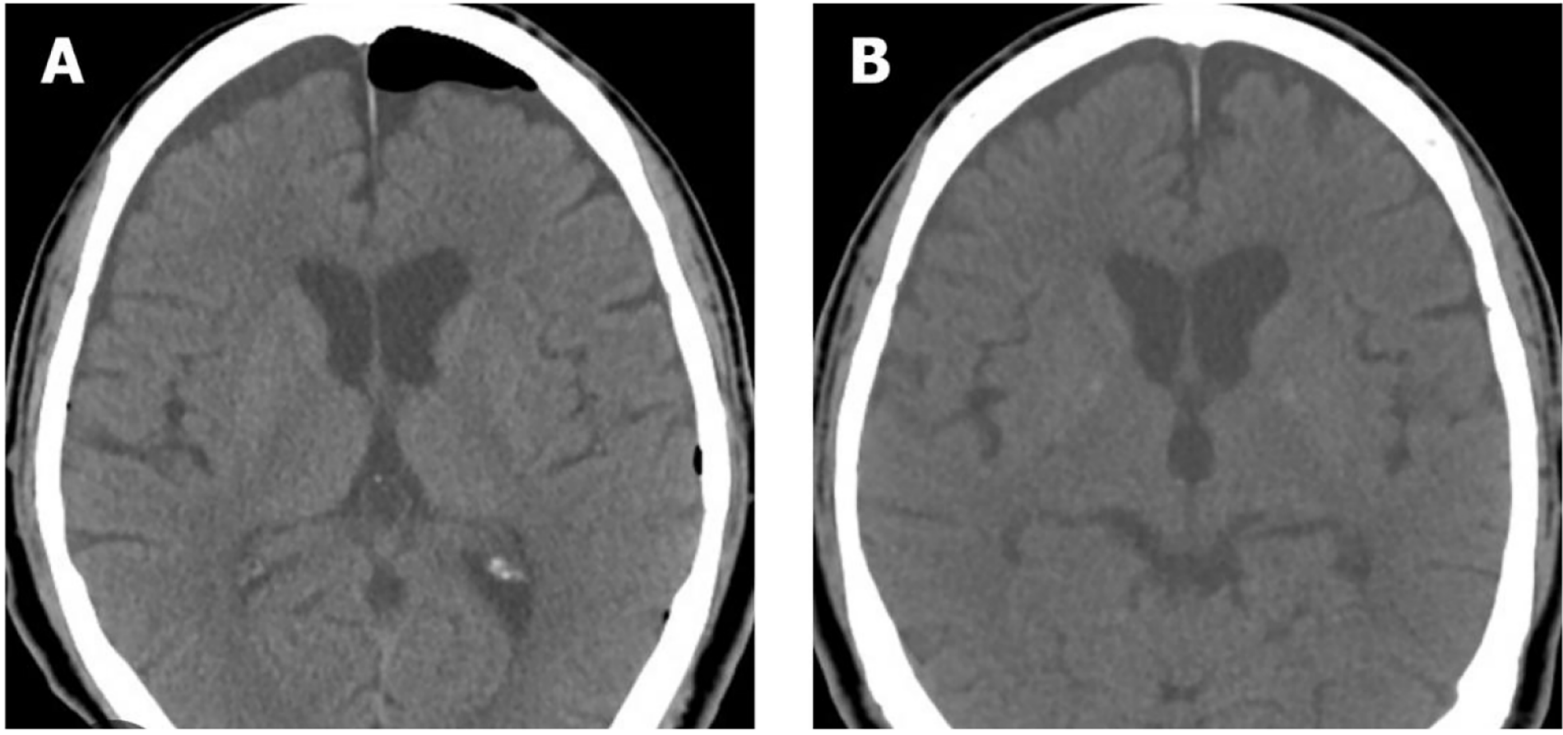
The brain computed tomography (CT) scan; A, before treatment; B, after treatment
